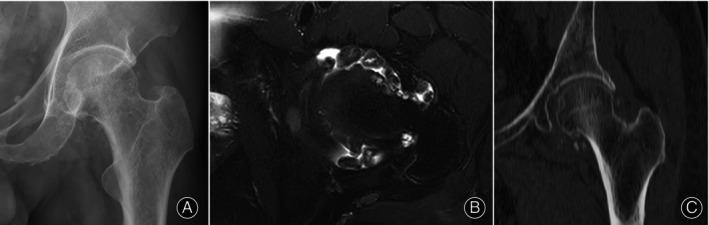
Radiographic appearance of hip synovial osteochondromatosis (left hip, PT‐5): (A) x‐ray, (B) magnetic resonance (MR) T2‐weighted image, and (C) computed tomography (CT) coronal reconstruction image.
